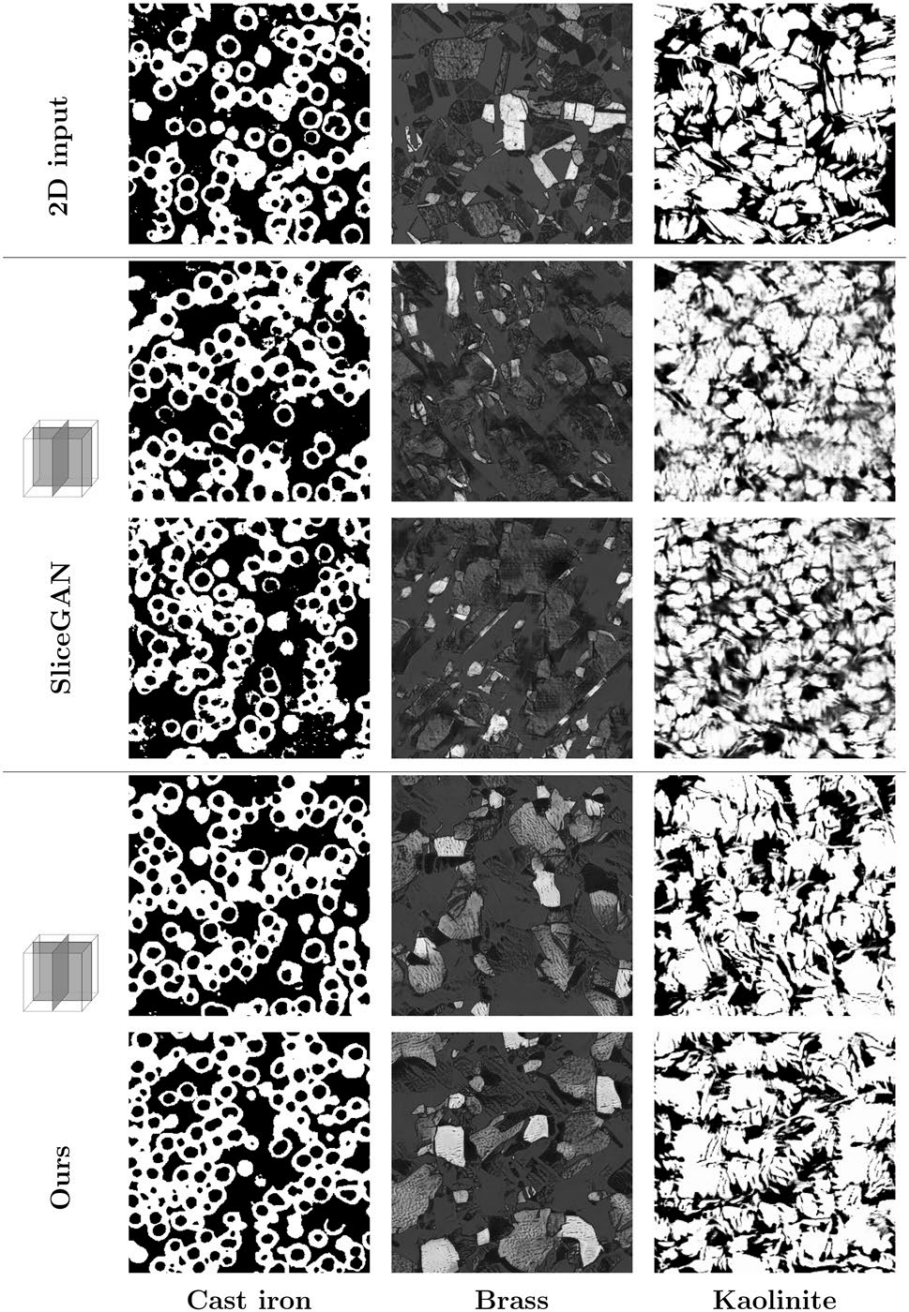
Visual comparison with non-CT data sources: cross-sections of 3D images produced by our method and SliceGAN, presented alongside their respective 2D training images. This figure includes images of cast iron, brass, and kaolinite clay mineral.
